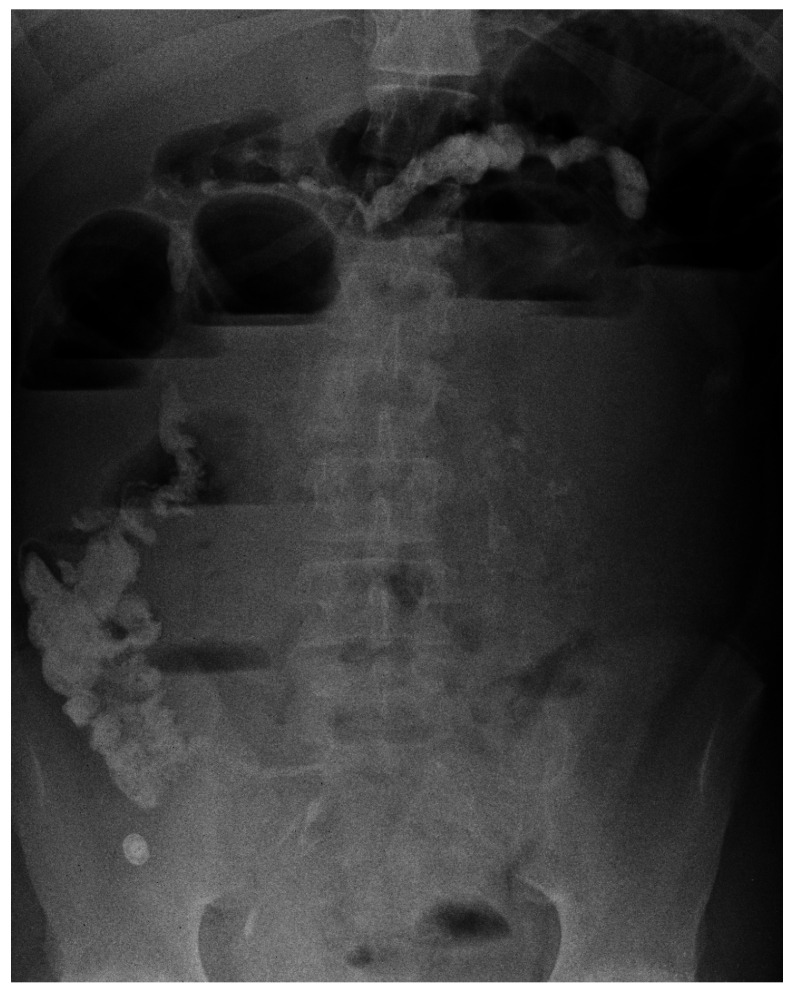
Abdominal X-ray and signs of intestinal obstruction.
